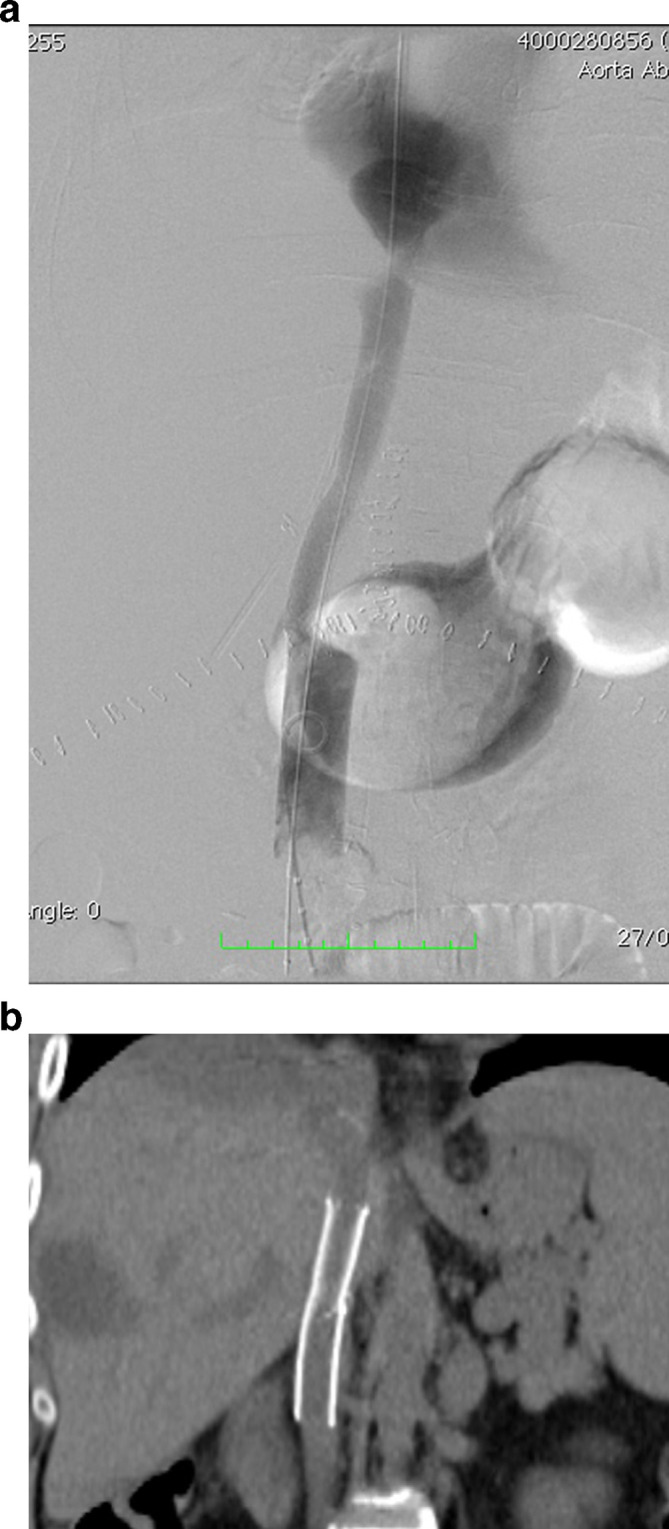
(a) Controlvenography shows revascularization with adequate flow towards the right atrium. (b) Abdomen CT with coronal reconstruction shows stent placement in the IVC.
